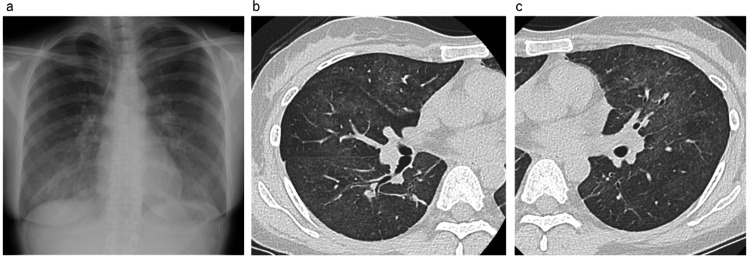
Chest radiography and high-resolution computed tomography (HRCT) of Case 1 on admission a: Chest radiography showing bilateral diffuse ground-glass opacity; b, c: HRCT showing bilateral diffuse ground-glass opacities with a subpleural sparing pattern
